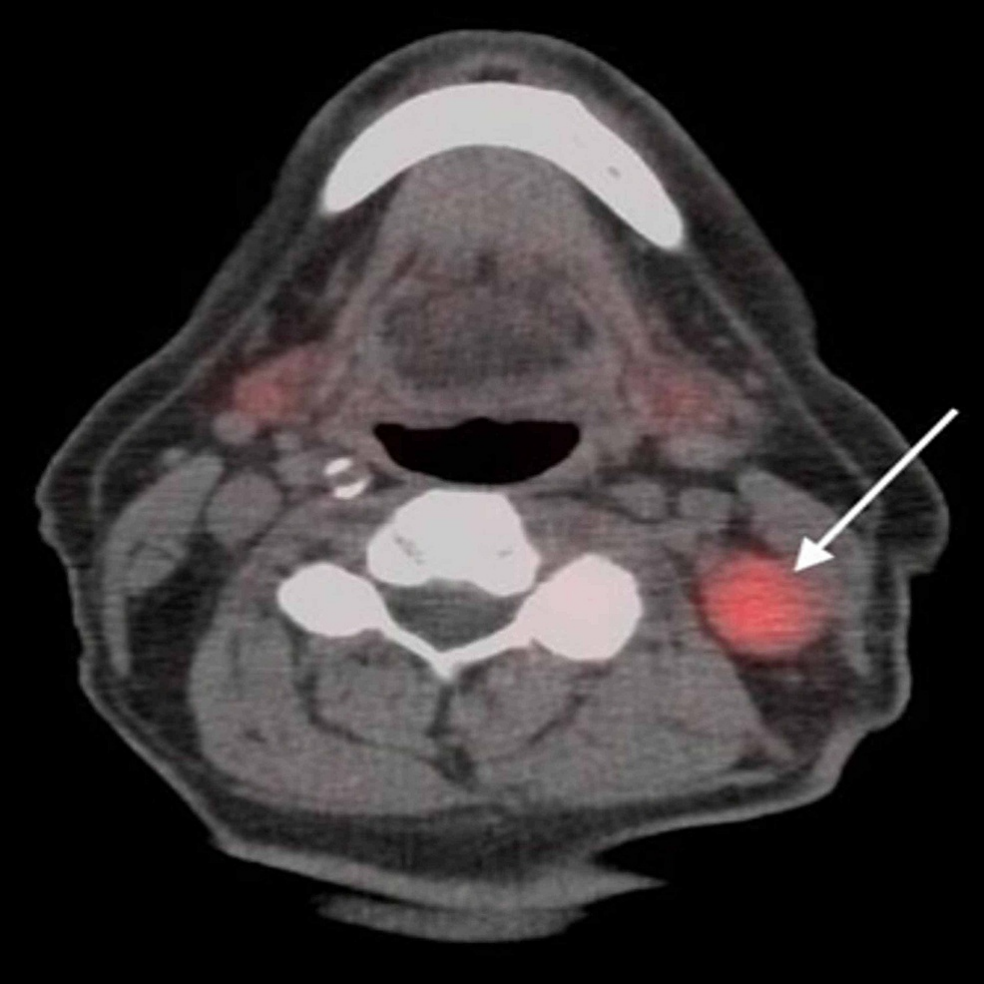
FDG-PET-CT axial view demonstrating increased uptake in the enlarged left upper jugular (posterior) lymph node FDG: fludeoxyglucose, PET: positron emission tomography, CT: computed tomography
